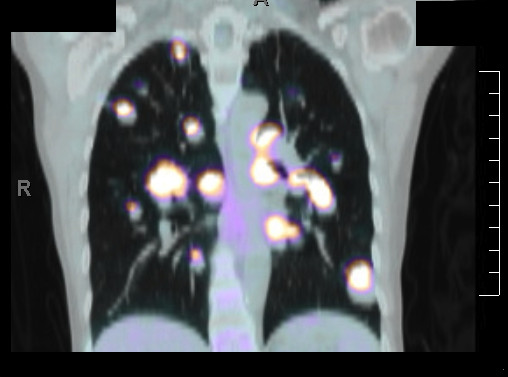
PET/CT scan demonstrating metastatic disease before any systemic therapy was initiated.
